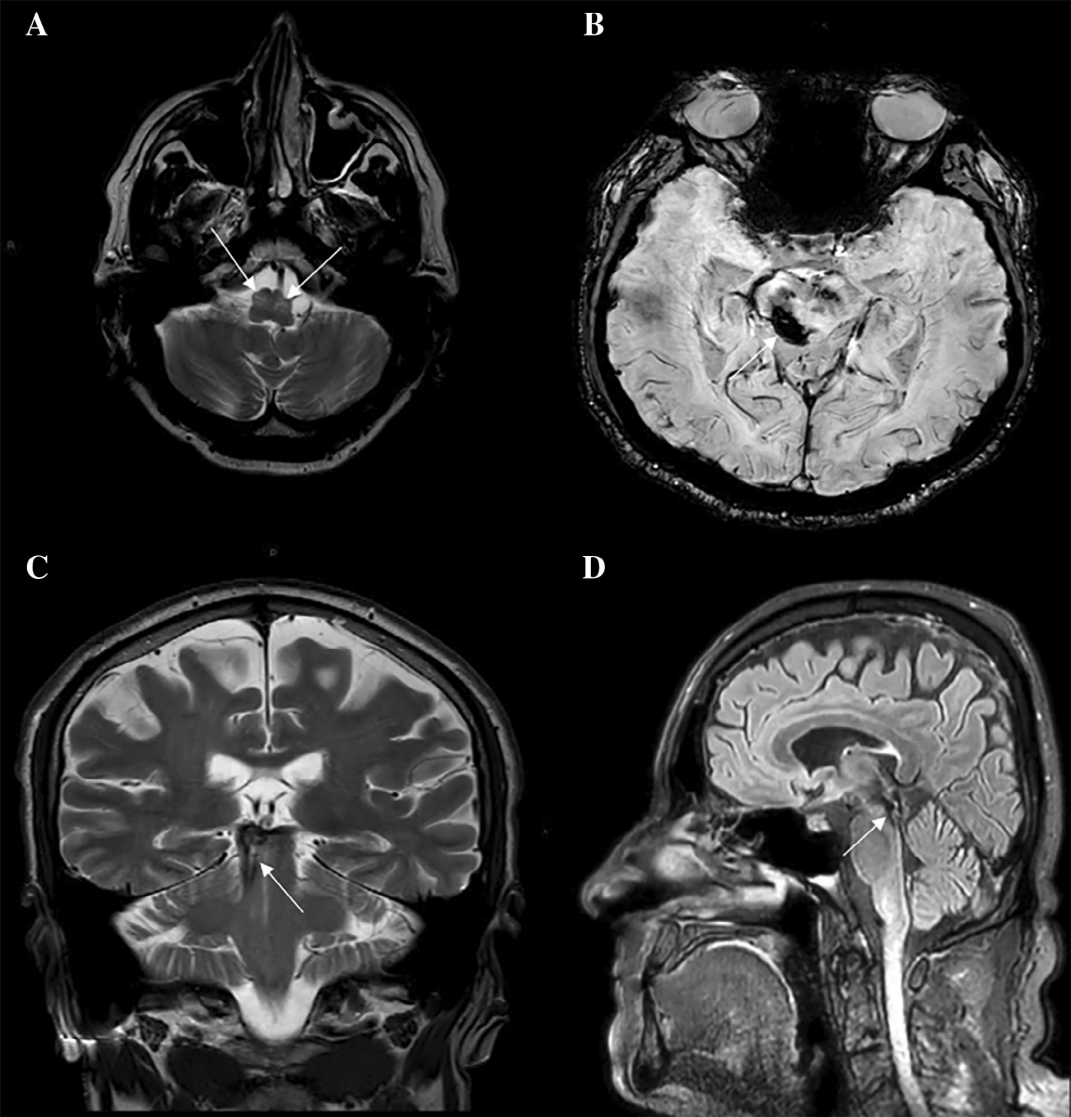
Magnetic resonance imaging findings. (A) Axial T2‐weighted bilateral hypertrophic olivary degeneration (arrows). (B,C) Susceptibility weighted imaging sequence and coronal T2‐weighted showed hemosiderin accumulation in right midbrain, superior cerebellar peduncle and pons. (D) Sagittal fluid attenuated inversion recovery (FLAIR) showed clear involvement of Guillan‐Mollaret triangle but no signs of cerebellar atrophy.
